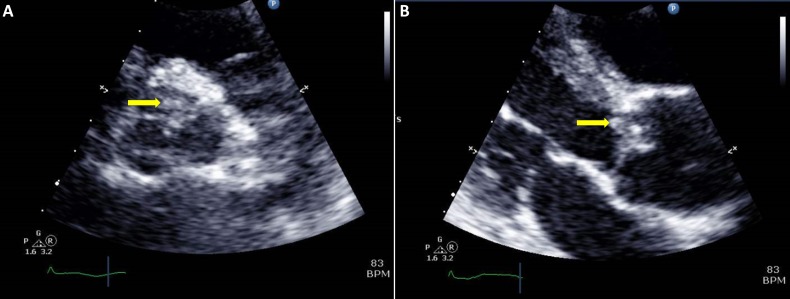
Transthoracic echocardiography Transthoracic echocardiography (A-B) showing tiny finger-like projections (yellow arrows) over the aortic valve, suggestive of vegetation over the aortic valve
